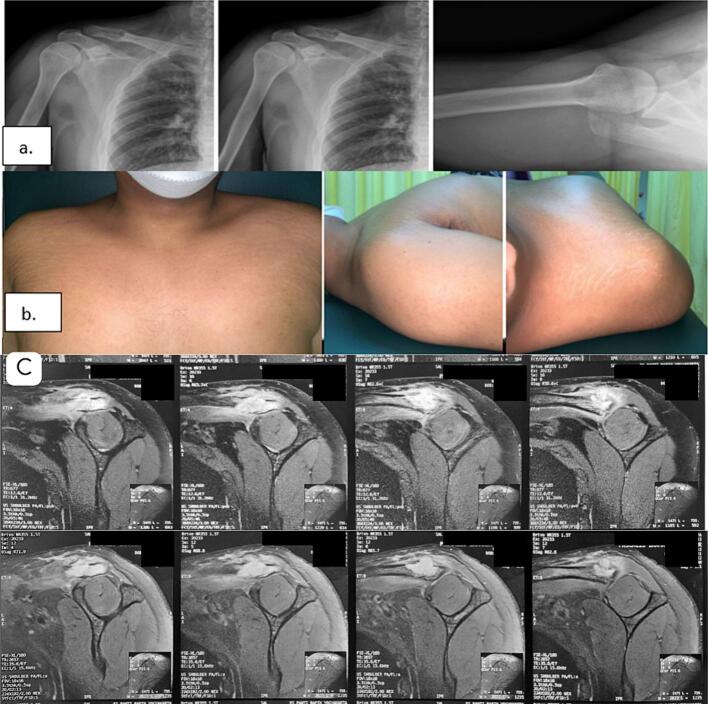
a. Pre-operative radiograph showing lytic lesion on distal clavicle; b. asymmetrical shoulder from; c. MRI with contrast enhancement.
